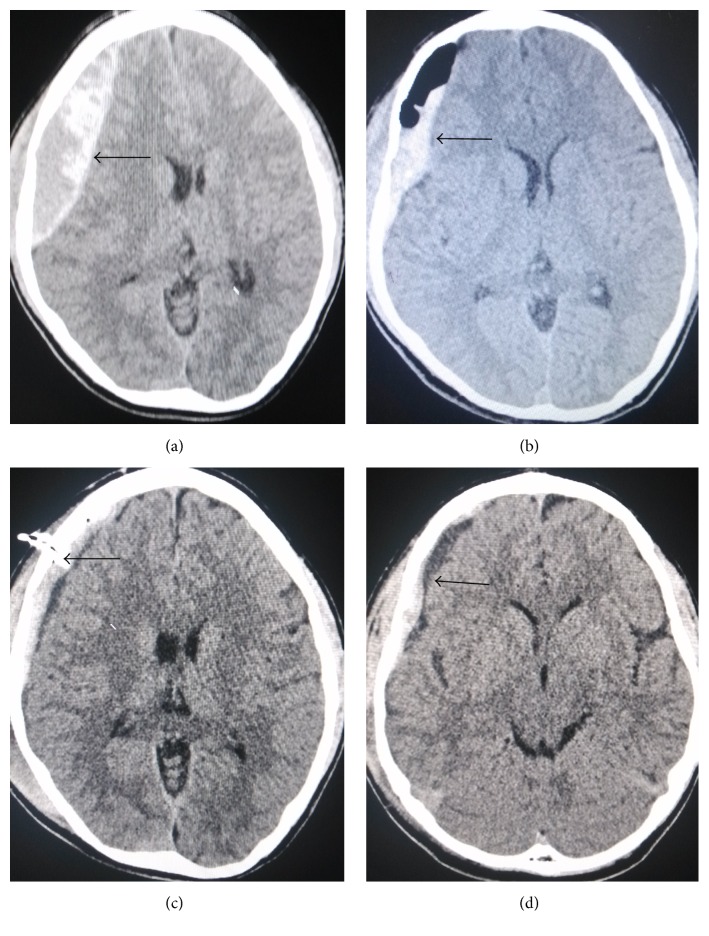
(a)–(d) Head CT images showing minimally invasive drainage treatment of acute epidural hematoma at the right temporal-parietal region, prior to and after surgery. (a) Head CT scan image prior to surgery. Hematoma volume was approximately 80 mL, midline shift. (b) Head CT scan image immediately after surgery; hematoma volume was markedly reduced with a residual amount of approximately 30 mL. (c) At 3 days after surgery, most of the hematoma has been drained; arrow indicates a drainage tube. (d) Five days after surgery, hematoma was almost cleared, and the drainage tube was pulled off.
